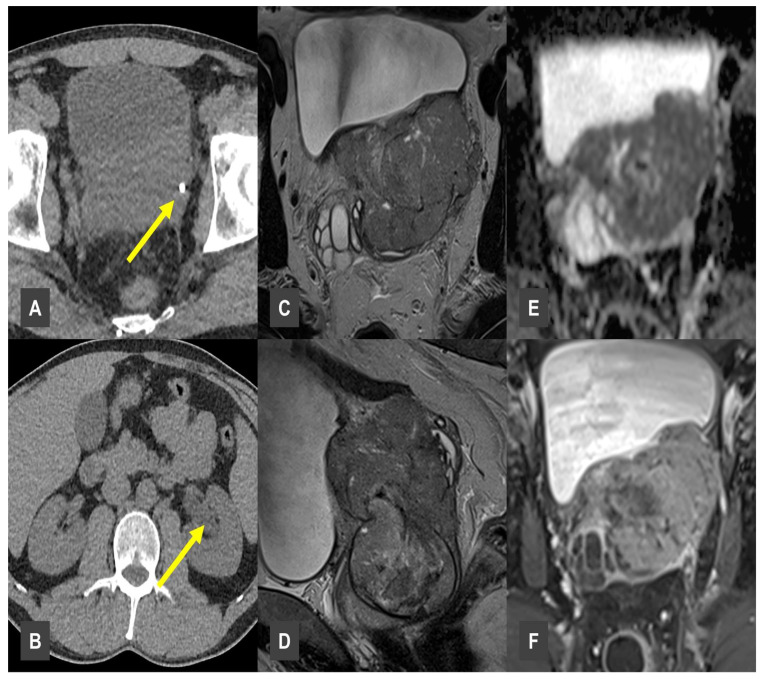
Prostate infiltrating Sarcoma. (A,B) CT showing urolithiasis with obstructive changes (yellow arrows). (C,D) MRI demonstrating a large T2w hypointense pelvic mass. (E) Diffusion-weighted MRI showing diffusion restriction within the pelvic lesion, suggestive of high cellularity. (F) Lesion expressed vivid contrast enhancement.
